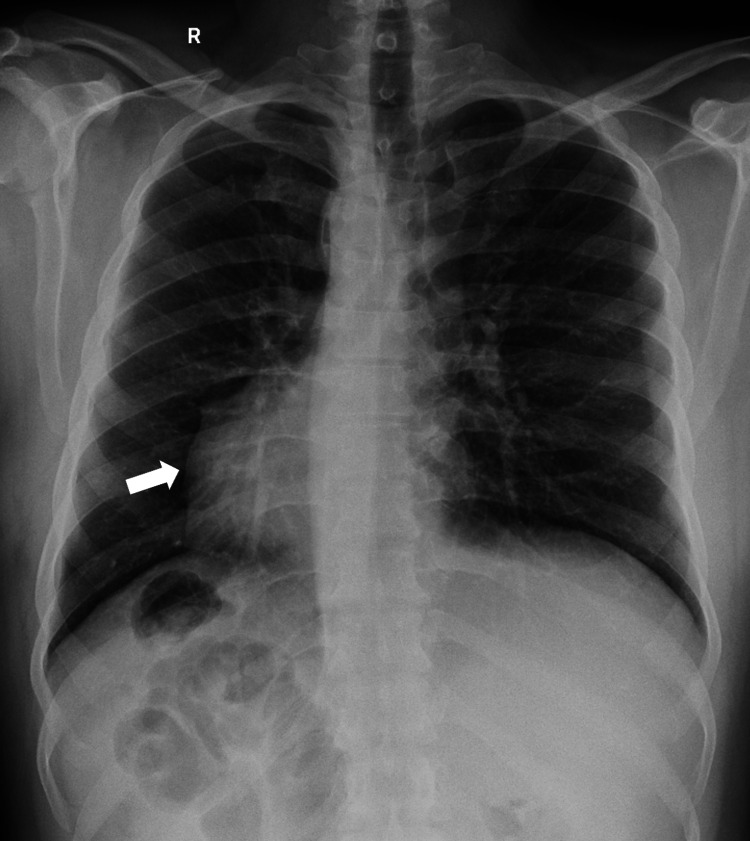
Chest X-ray showing cardiac shadow at the right
